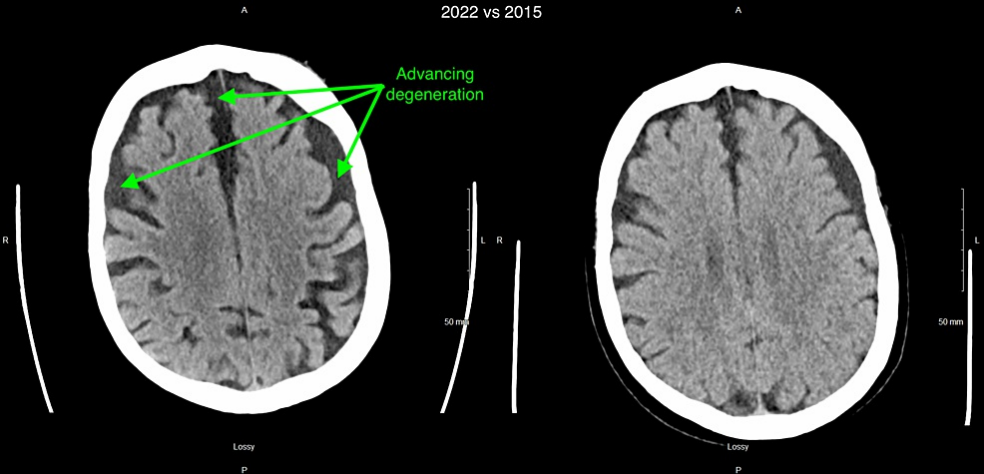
Computed tomography (CT) without contrast of the head 2022 vs 2015 (3) 2022 scan is on the left while 2015 scan is on the right
